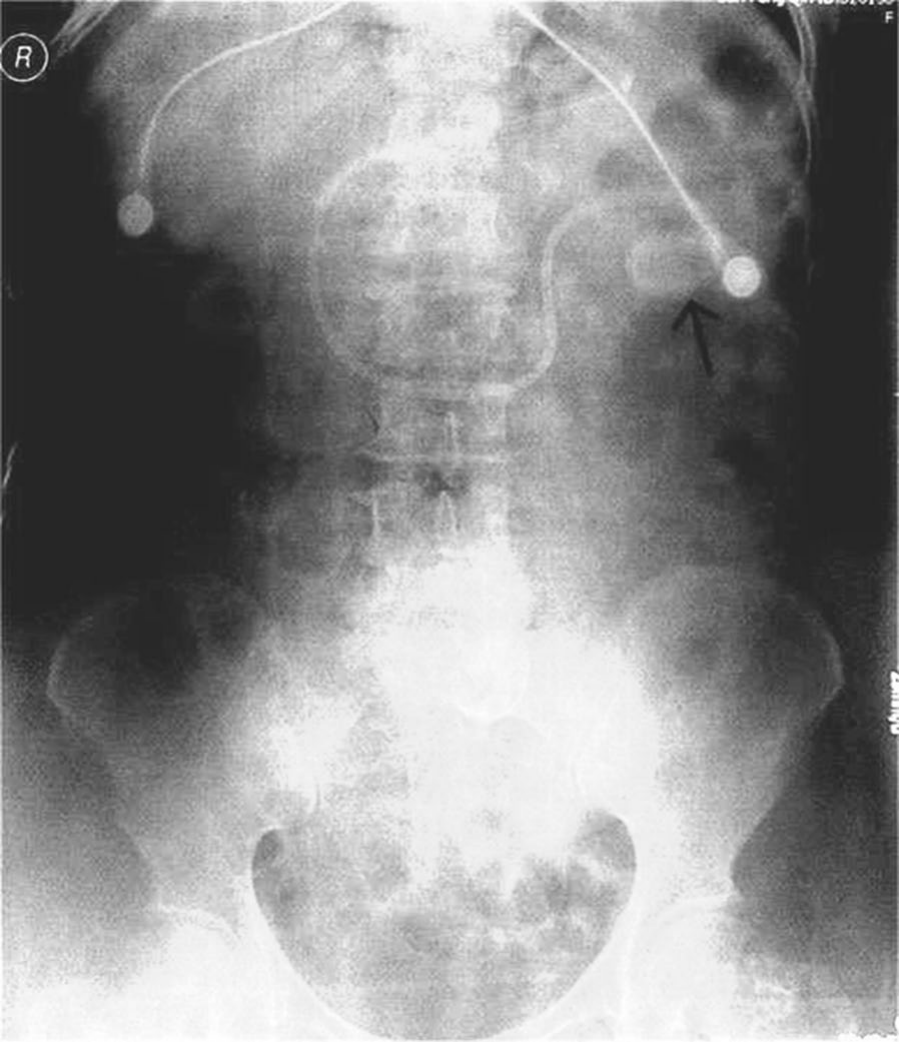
Abdomen and chest X-ray. The *arrow* indicates the tip of the nasointestinal tube within the jejunum
